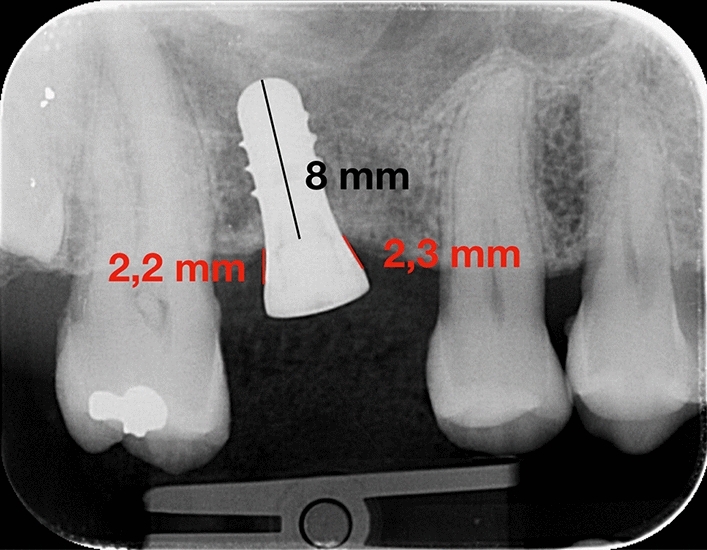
Example of X-ray measurement for MBL: 8 mm was the length of the implant used for calibration, while 2.3 and 2.2 mm show the mesial and distal MBL measurement, respectively
